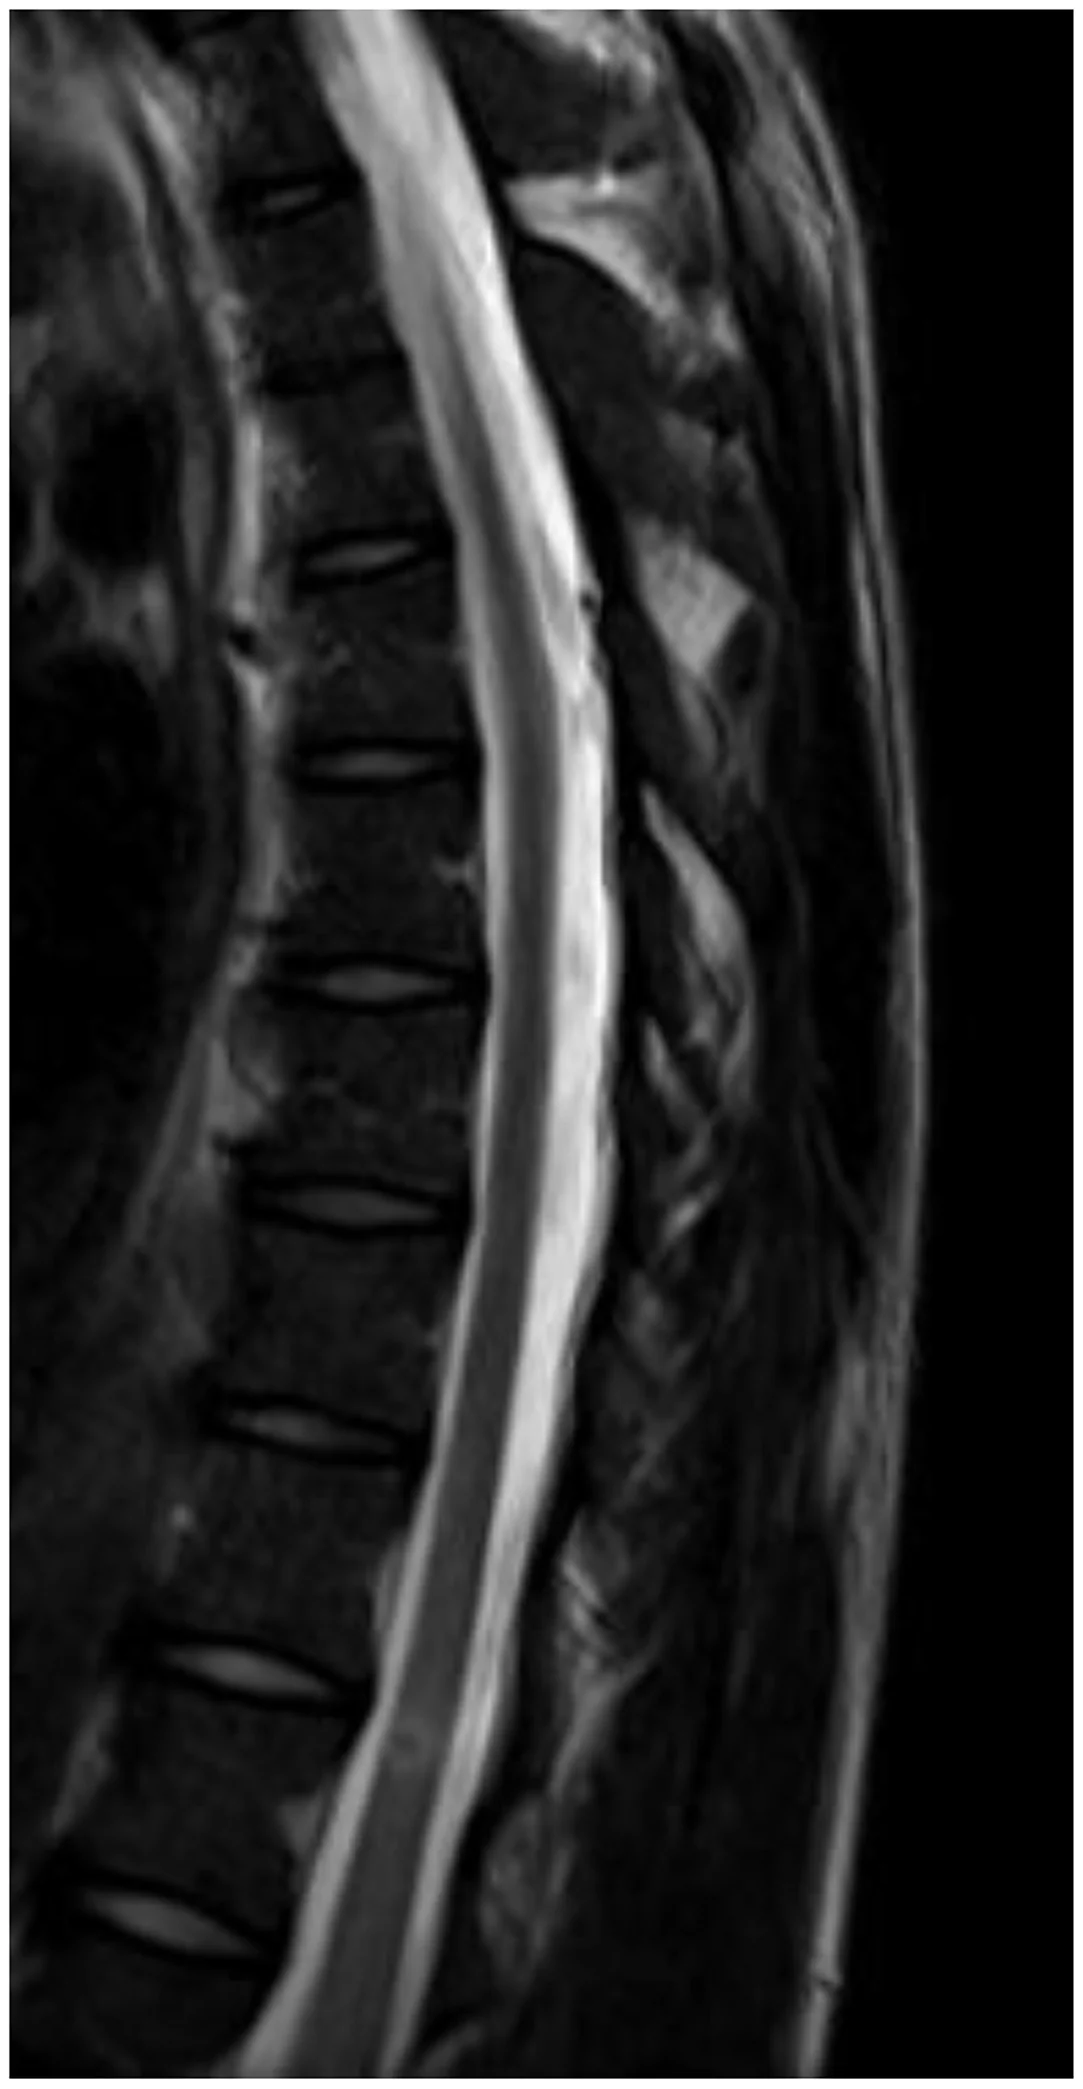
Sagittal T2-weighted MRI obtained after treatment demonstrates a marked reduction in the size of the previously described nodular intramedullary lesion at the T10-T11 level. The associated vasogenic edema has completely resolved, with normalization of the adjacent cord signal. These findings are consistent with a significant radiological response to the institute therapy.
